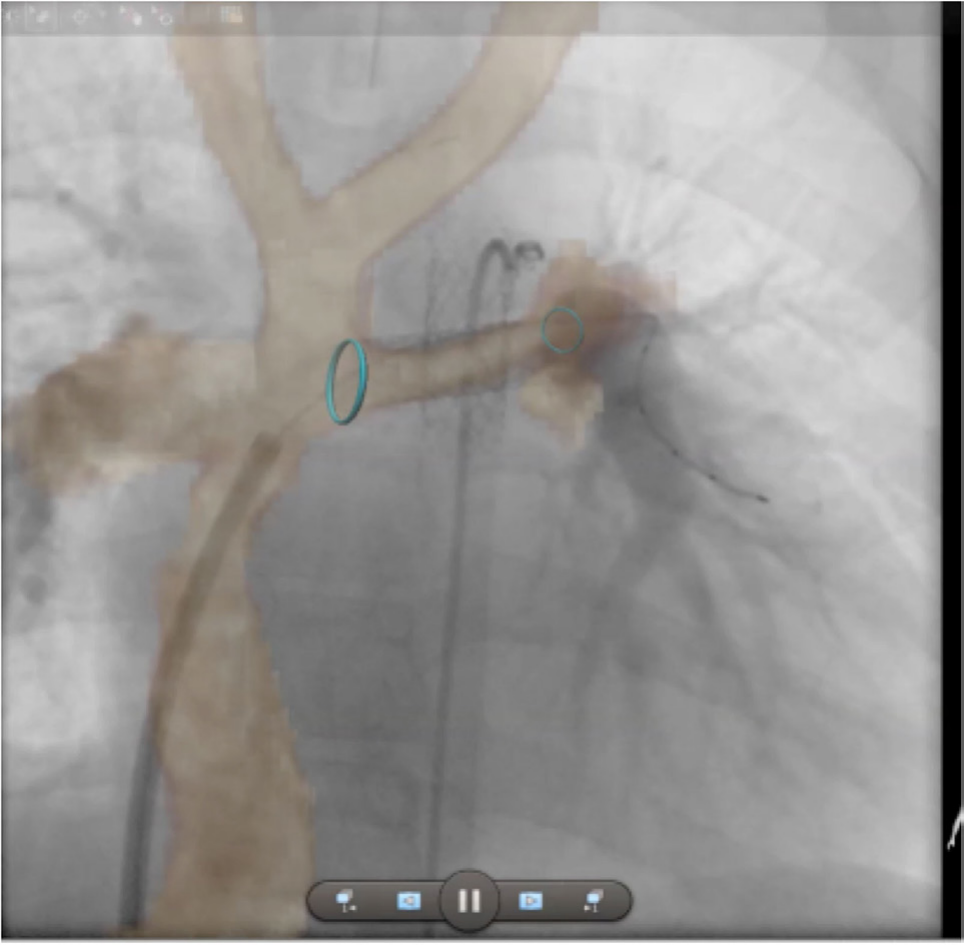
Augmented fluoroscopy. Three-dimensional whole heart MR images are fused with angiographic images to augment procedural guidance. In this case, the left pulmonary artery in a child with a Fontan circuit has been stented. The optimal angulation for imaging is predetermined using the MR dataset. Blue ring markers on the MRI dataset are used to guide the positioning of the stent. The images show good registration on the angiogram after stenting has taken place.
